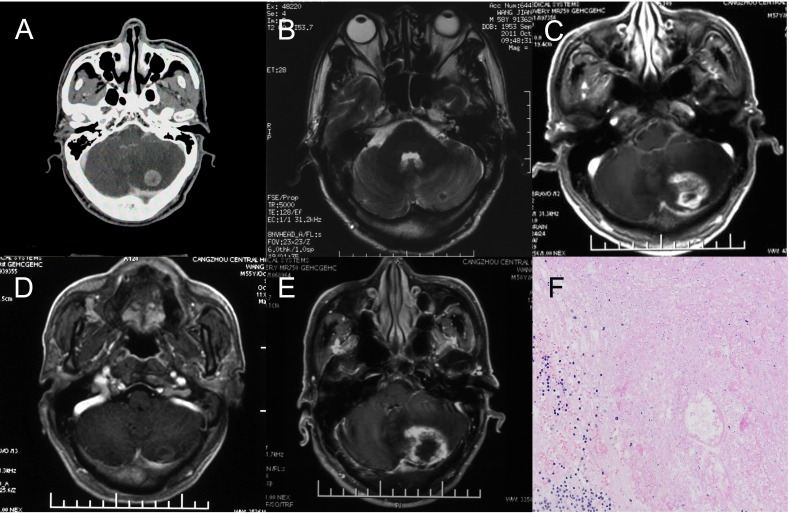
One case of brain necrosis recurrence after bevacizumab discontinuation A. The metastasis lesion before Cyberknife treatment. B. 3 months after Cyberknife treatment. The lesion significantly reduced, almost disappeared. C. 14 months after Cyberknife treatment. The brain necrosis occurred and the bevacizumab was used. D. the patient received bevacizumab at 5 mg/kg, q3w× 3cycles.A brain MRI scan showed significant improvement in the necrosis and then the bevacizumab discontinued (nearly disappeared). E. 5 Months after the bevacizumab discontinuation, MRI scans showed a large area with enhancement and edema at the original treatment site. The diagnosis by radiologists was cerebral necrosis relapse. F. A Local excision was treated and the pathological examination confirmed radiation brain necrosis.
